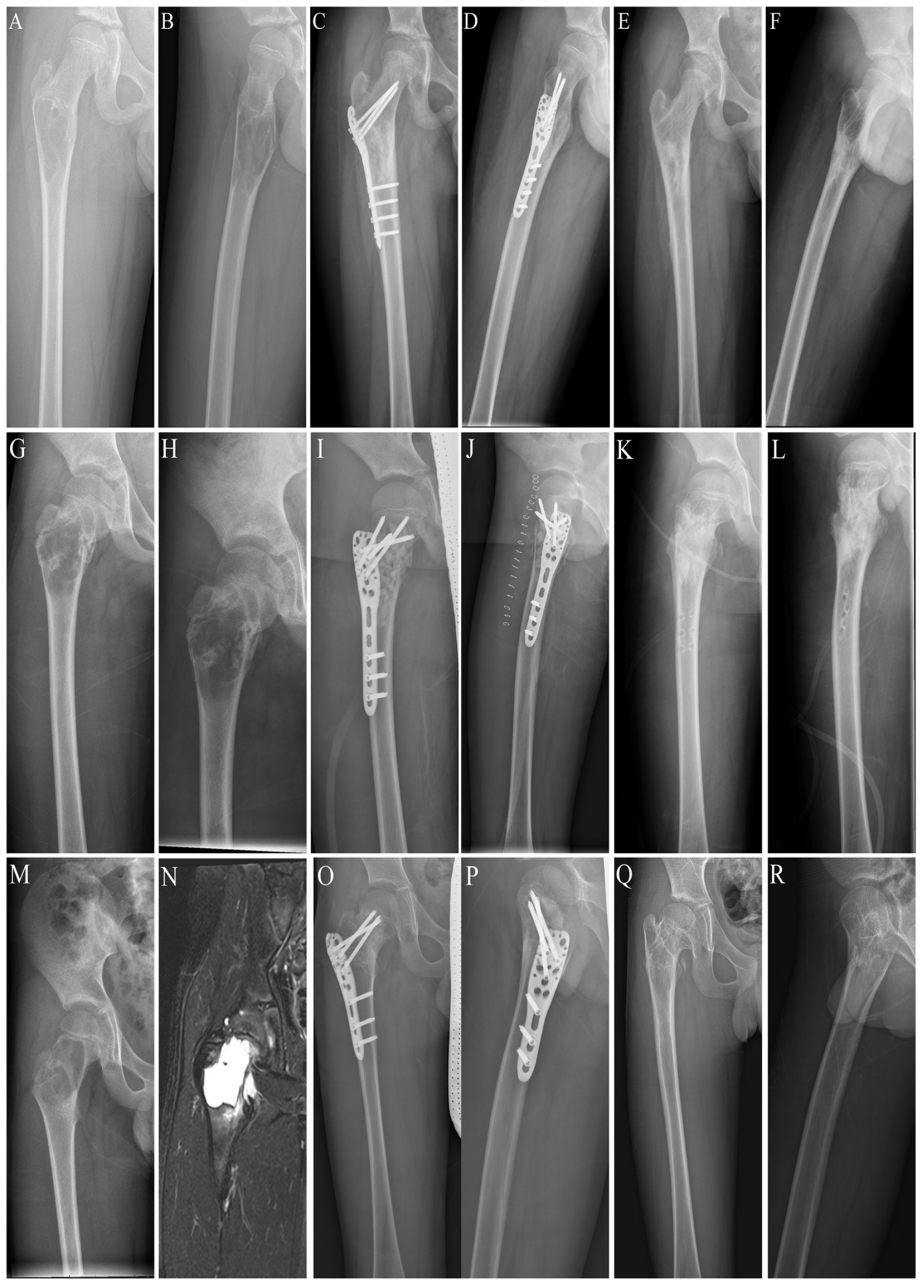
Three different locations of benign lesions of the proximal femur. (A) Preoperative posteroanterior x-ray of a subtrochanteric lesion. (B) Preoperative lateral x-ray image of a subtrochanteric lesion. (C) Postoperative posteroanterior x-ray image of the internal fixation for a subtrochanteric lesion. (D) Postoperative lateral x-ray image of the internal fixation for a subtrochanteric lesion. (E) Posteroanterior x-ray image after removing the locking plate and screws from the subtrochanteric lesion. (F) Lateral x-ray image after removing the locking plate and screws from the subtrochanteric lesion. (G) Preoperative posteroanterior x-ray image of an intertrochanteric lesion. (H) Preoperative lateral x-ray image of an intertrochanteric lesion. (I) Postoperative posteroanterior x-ray image of internal fixation for an intertrochanteric lesion. (J) Postoperative lateral x-ray image of the internal fixation for an intertrochanteric lesion. (K) Posteroanterior x-ray image after removal of the locking plate and screws from the intertrochanteric lesion. (L) Lateral x-ray image after removing the locking plate and screws from the intertrochanteric lesion. (M) Preoperative posterior x-ray images of the intertrochanteric part and the femoral neck lesion. (N) Preoperative lateral x-ray image of the intertrochanteric segment and the femoral neck lesion. (O) Postoperative posteroanterior x-ray image of internal fixation for the intertrochanteric part and the femoral neck lesion. (P) Postoperative lateral x-ray image of internal fixation for the intertrochanteric part and the femoral neck lesion. (Q) Posteroanterior x-ray image of the intertrochanteric part and femoral neck lesion after removing the locking plate and screws. (R) Lateral x-ray image after removing the locking plate and screws from the intertrochanteric and femoral neck lesions.
